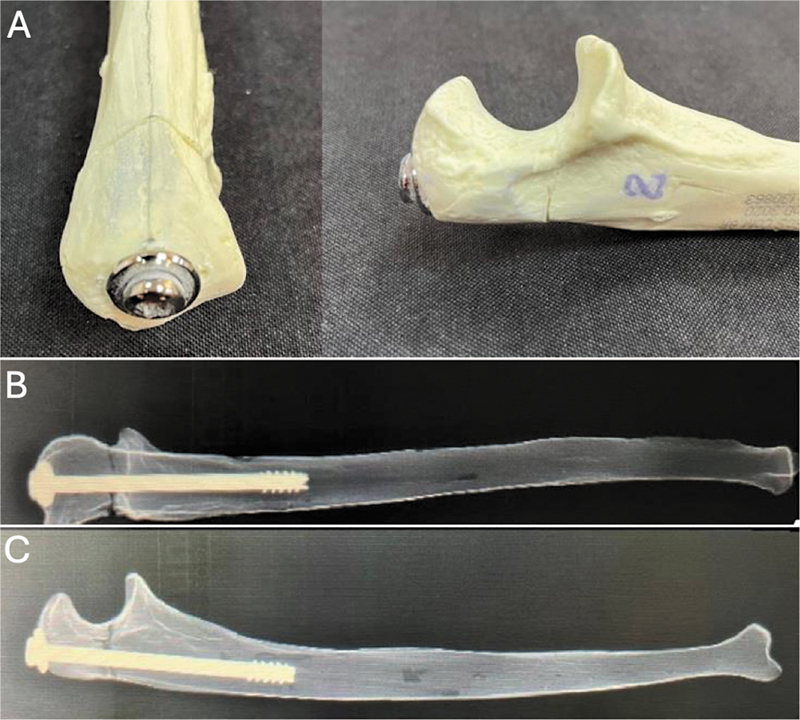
Intramedullary (IM) screw fixation. (A) Osteotomy fixation with longitudinal insertion of a cannulated screw into the medullary canal. (B) Anteroposterior and lateral (C) radiographs of radiopaque ulnae after osteotomy fixation with an IM screw.
